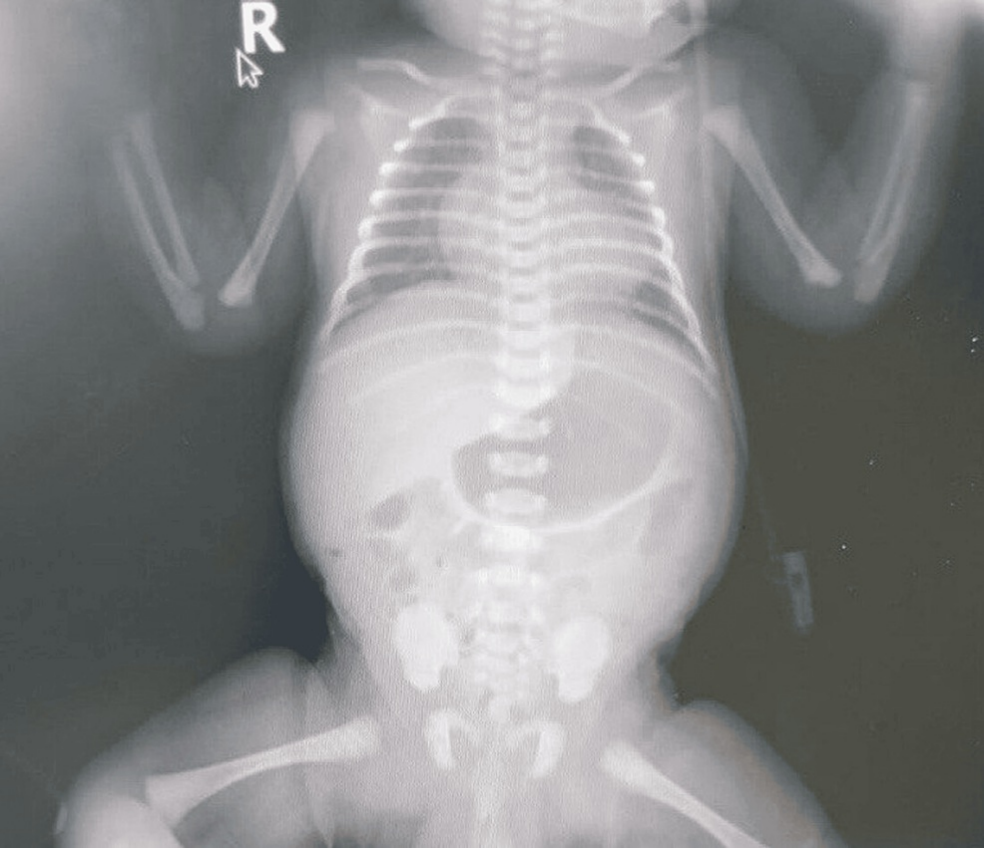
Chest X-ray on day five revealed a marked resolution of pulmonary haziness and a notable reduction in cardiac silhouette size, indicating improvement in the patient’s condition.
